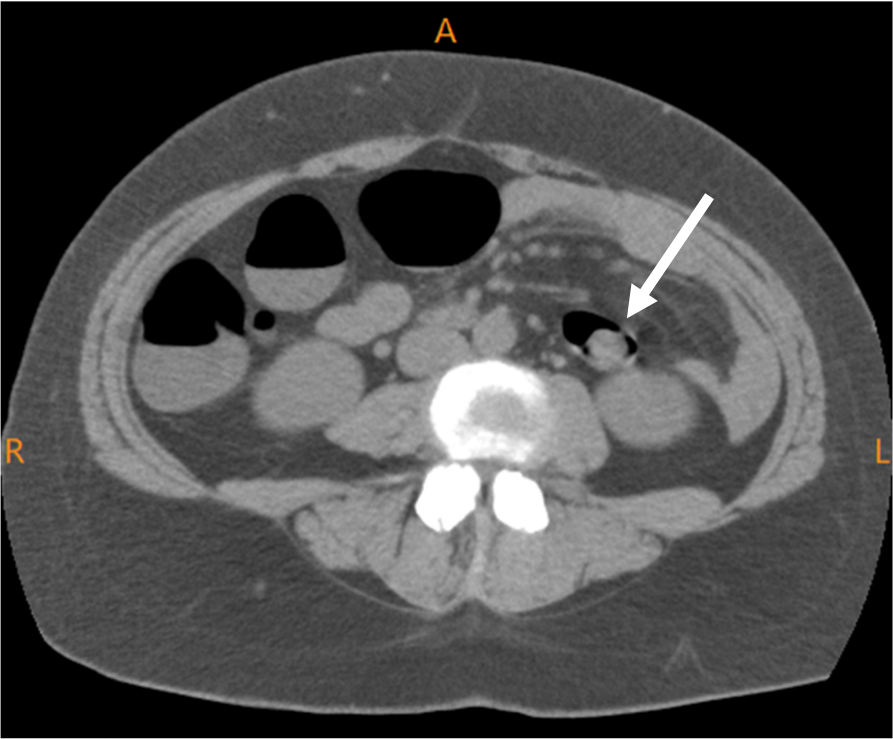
Computed tomography. Imaging performed before the initial endoscopic resection shows wall thickening in the descending colon but no enlarged lymph nodes or distant metastasis
